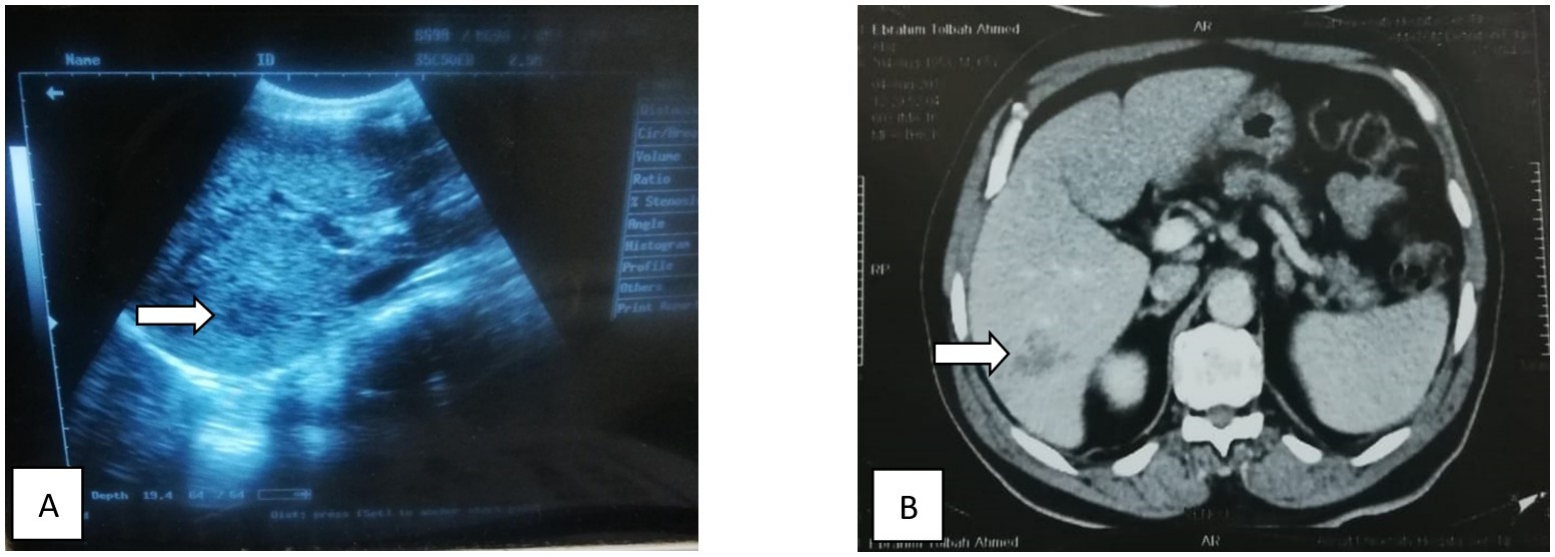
A. Ultrasound image; B. Abdominal CT image of a hepatic focal lesion (HFL) caused by fascioliasis in the right lobe (white arrows).
